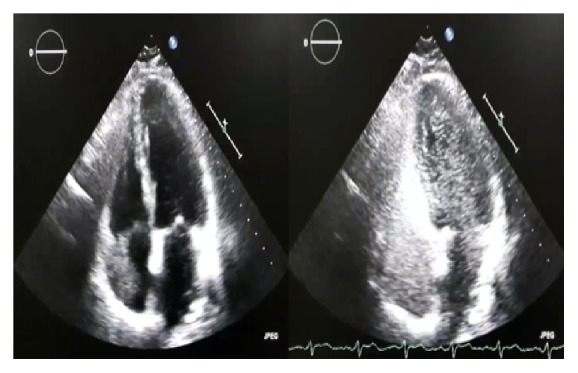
Contrast-enhanced transthoracic echocardiography showing contrasts' microbubbles in the left side of the heart.
